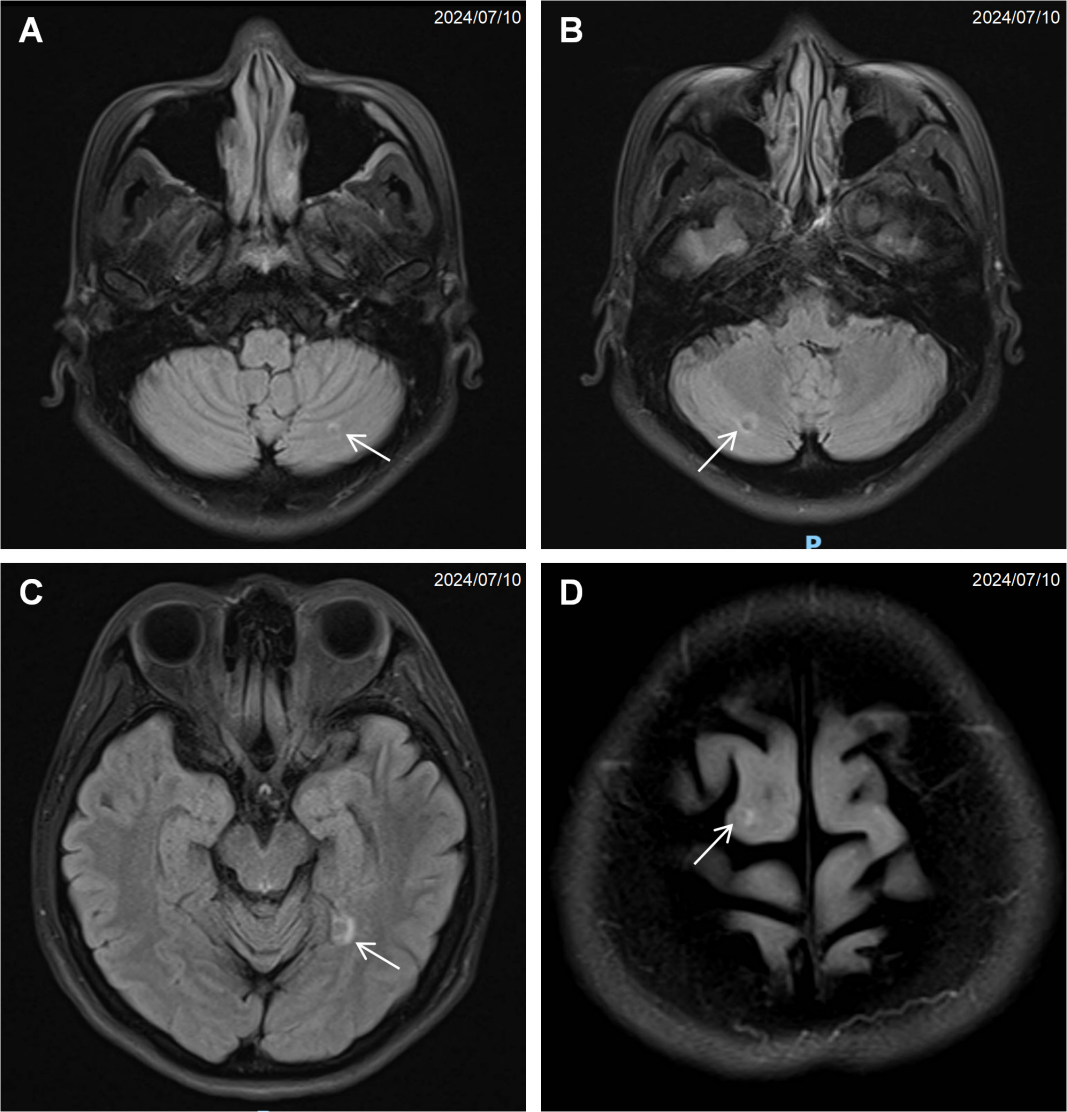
Magnetic resonance imaging (MRI) of the brain showing multiple small nodular signal abnormalities: (A) left cerebellar hemisphere (arrow), (B) right cerebellar hemisphere (arrow), (C) left temporal lobe (arrow), (D) right frontal lobe (arrow).
